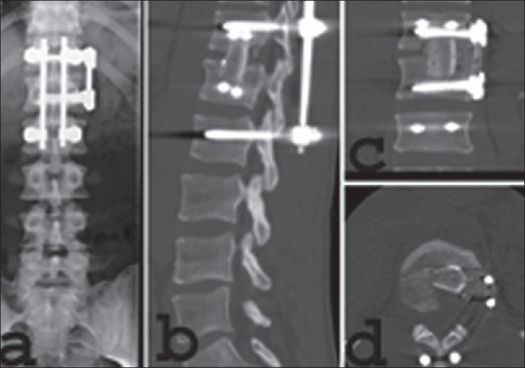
Posterior reduction and fixation followed by thoracoscopic anterior decompression and mono-segmental reconstruction using tricortical bone graft and anterior instrumentation. Plain X-ray AP (a) and lateral (b) coronal reconstruction C.T. (c) and axial CT scan (d) shows insitu posterior implant, adequate anterior decompression and anterior tricortical bone graft with anterior instrumentation
